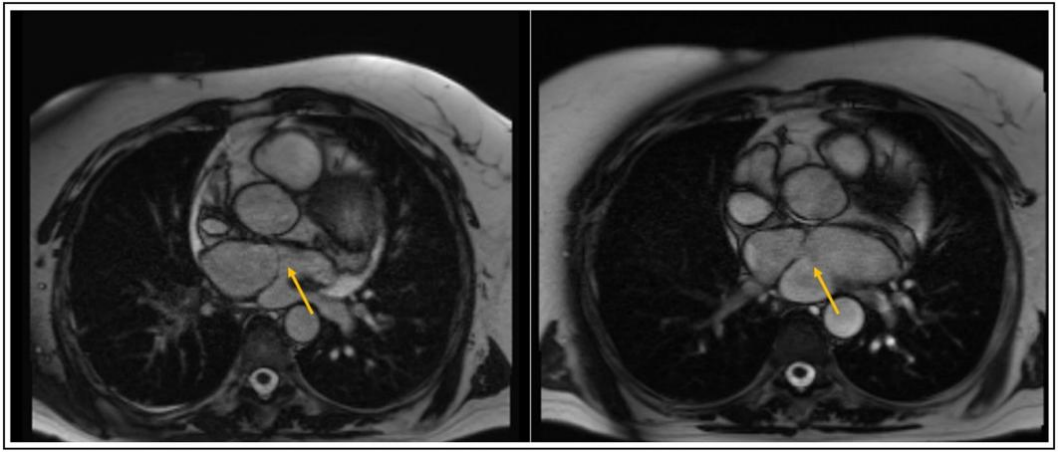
Magnetic resonance imaging of Patient 1 before and after percutaneous balloon dilatation. On the left, a large accessory chamber is seen with a small, restricted opening across the membrane to the left atrium. On the right, 18 months after percutaneous balloon dilatation, a decrease in the dimension of the accessory chamber is seen with an increased opening across the membrane to the left atrium.
